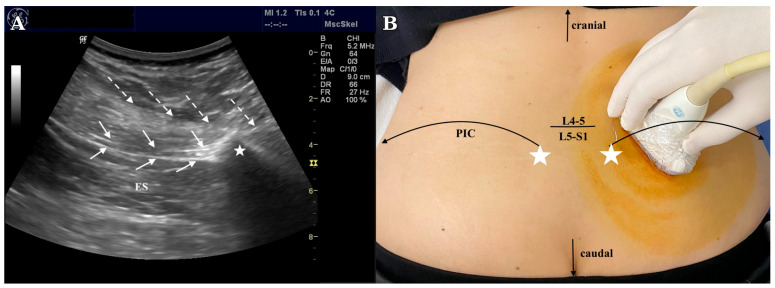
(A) Longitudinal ultrasonographic image demonstrating direct visualization of the SCN. The SCN (solid arrows) appears as a hypoechoic monofascicular structure coursing within the erector spinae muscle (ES) before passing between the thoracolumbar fascia (TLF; dashed arrows) and the posterior iliac crest (PIC; white star). Ultrasonographic visualization of the SCN. Adapted from Kiral et al. [6], with permission. (B) Patient positioning and ultrasound probe placement for longitudinal ultrasonographic examination of the SCN. The posterior superior iliac spine (PSIS; white stars), the PIC, and the L4–L5/L5–S1 levels are shown as surface anatomical landmarks to facilitate localization of the SCN. Cranial and caudal orientation are indicated.
